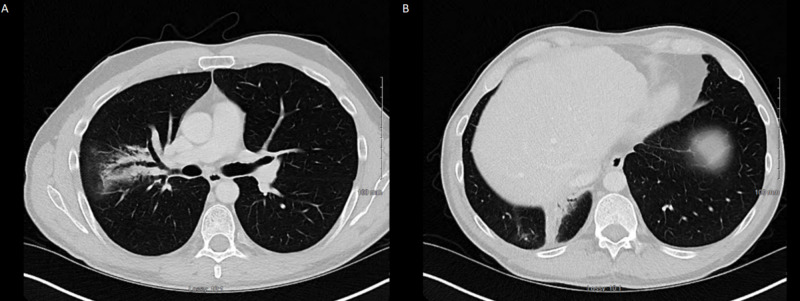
The figure shows the chest CT scan after a 14-month interval from his last SBRT treatment. It shows post-radiation changes and no evidence of progression. He also had no other sites of metastatic disease. (A) Shows radiation changes in the upper right lung. (B) Shows radiation changes in the lower right lung. SBRT, stereotactic body radiation therapy.
